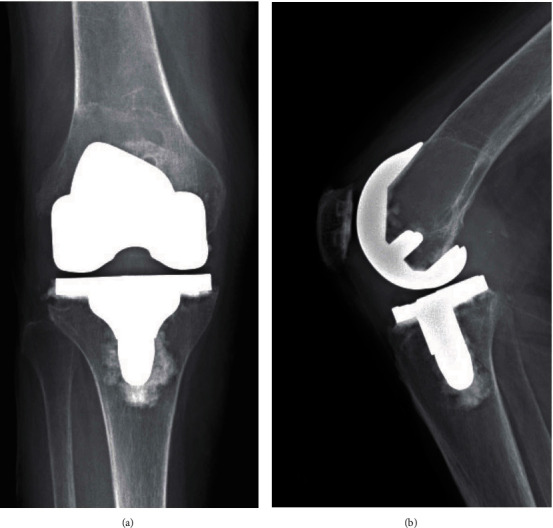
Right knee: (a) AP and (b) lateral radiographs at the time of presentation (February 2019).
